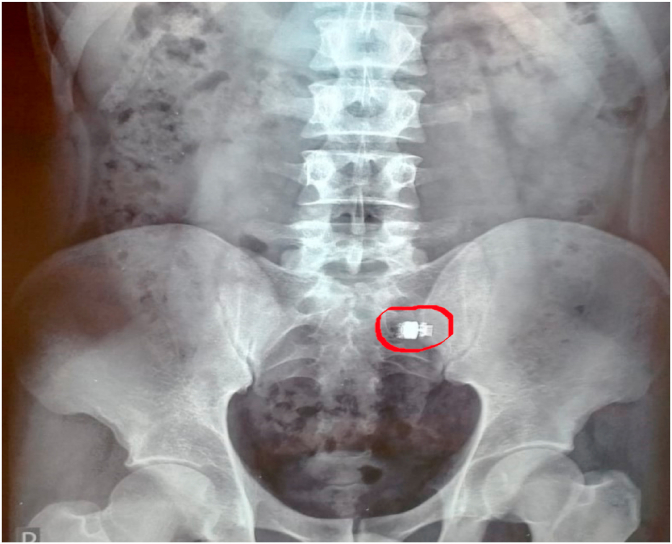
Image of capsule retention on an unprepared abdominal X-ray.
